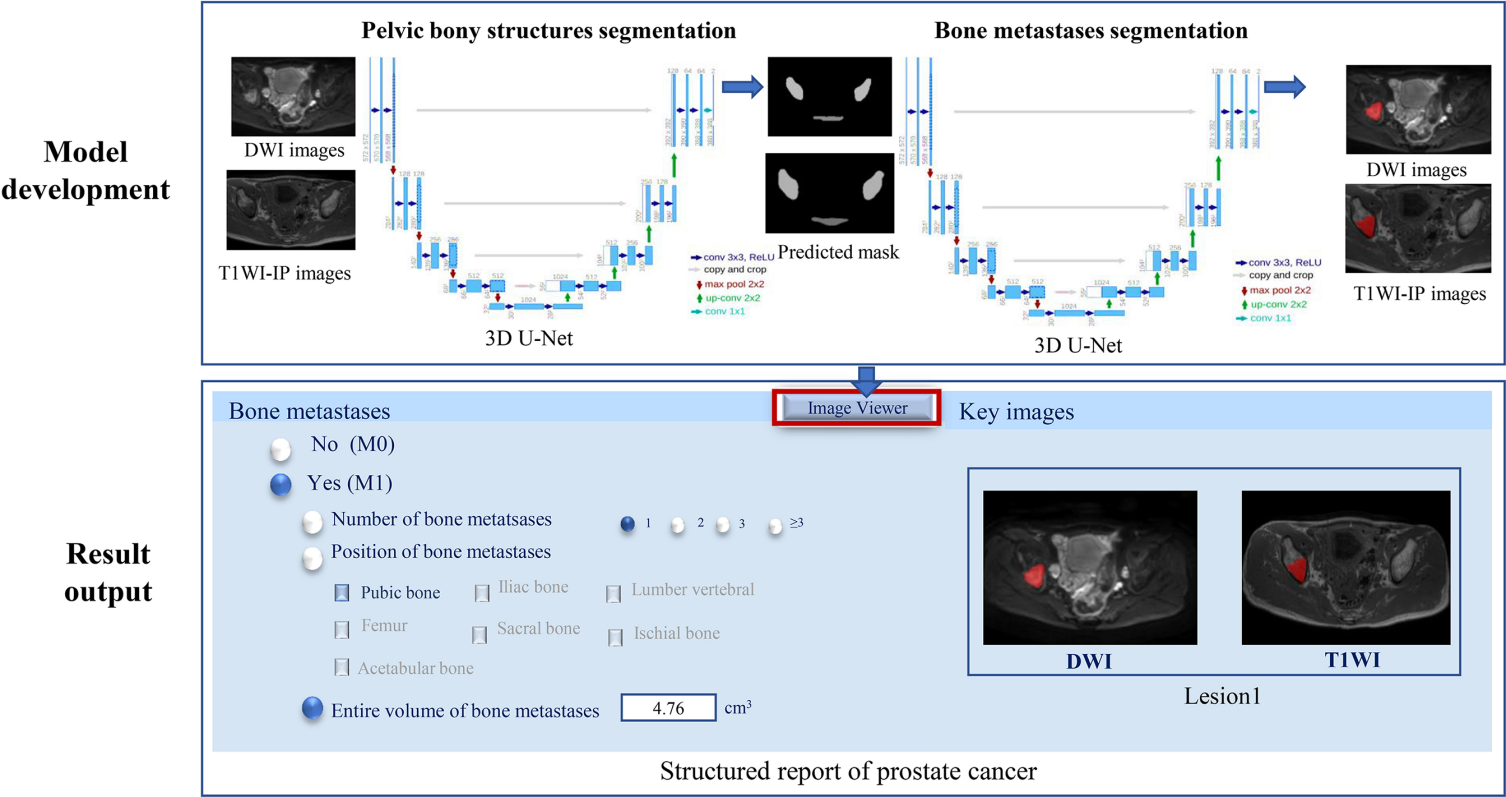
The two-step 3D U-Net for bone metastases segmentation on DWI and T1WI-IP images. T1WI-IP, T1W images obtained using the Dixon technique with in-phase.
